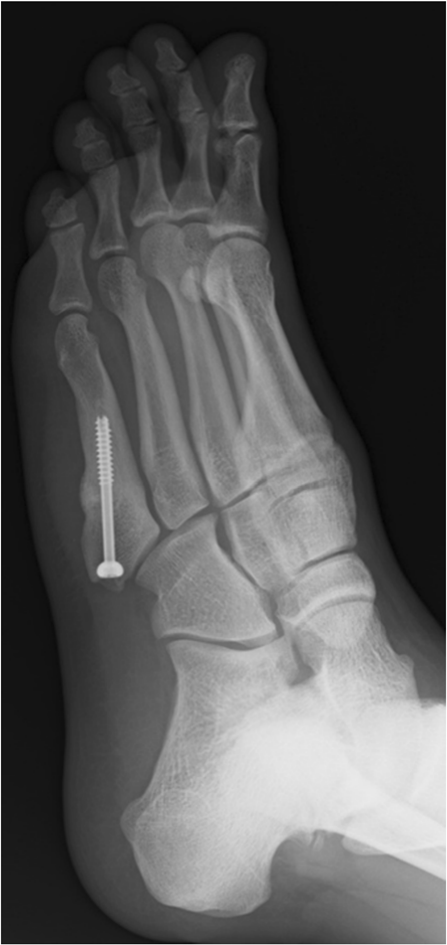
X-ray images from four directions to confirm bone union. Oblique radiograph at 45° internal rotation.
